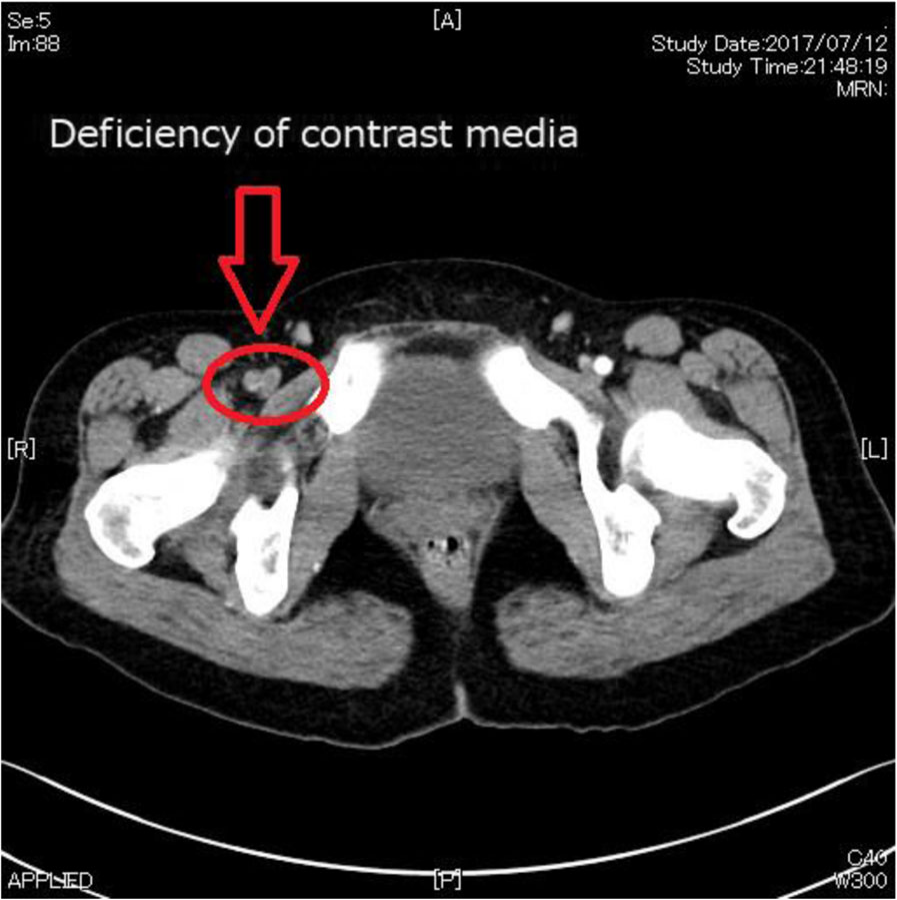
Contrast-enhanced computed tomography (CT) showing a deficiency of contrast media at the right femoral artery
